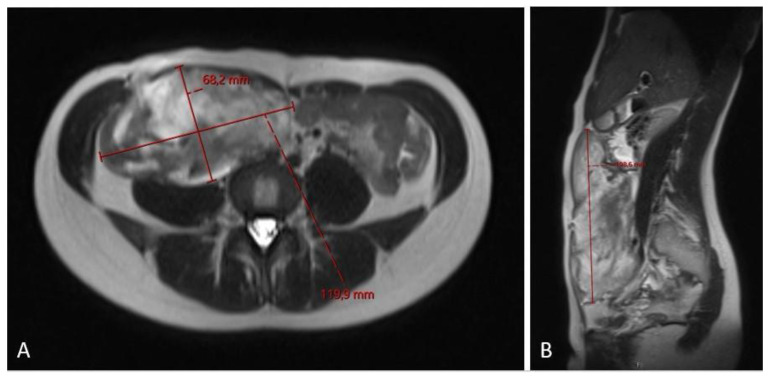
MRI scan ((A)—axial plane; (B)—sagittal plane) after 3 cycles of chemotherapy showing no effect of the treatment and slight progression in the craniocaudal tumor size (12.0 × 6.6 × 20.0 cm).
